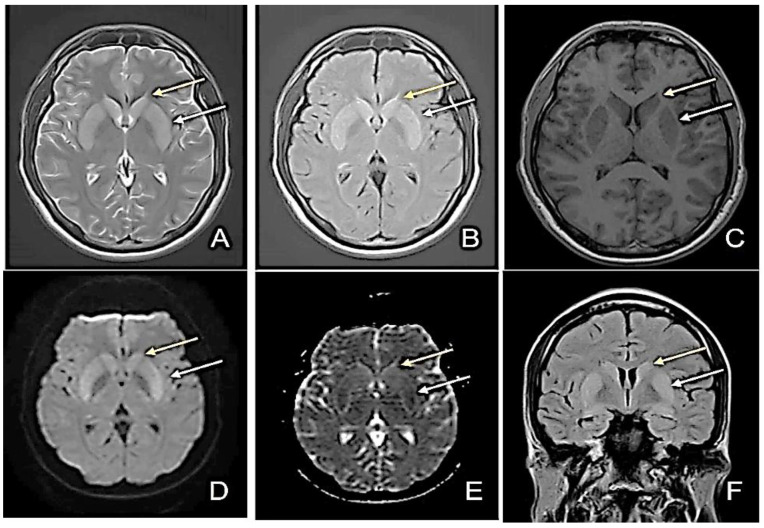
First Magnetic Resonance Imaging (MRI)—day 1 at the admission to our clinic (D1). (A) Axial T2-weighted image sequence: slightly increased bilateral putaminal and caudate nucleus signal; (B) Axial FLAIR sequence: increased bilateral putaminal and caudate nucleus signal; (C) Axial T1-weighted image sequence: slightly decreased bilateral putaminal and caudate nucleus signal; (D) Diffusion-weighted image sequence (DWI); (E) Apparent diffusion coefficient map (ADC): moderate bilateral putaminal restricted diffusion; (F) Coronal FLAIR image: slightly increased bilateral putaminal (white arrow) and caudate nucleus signal (yellow arrow).
